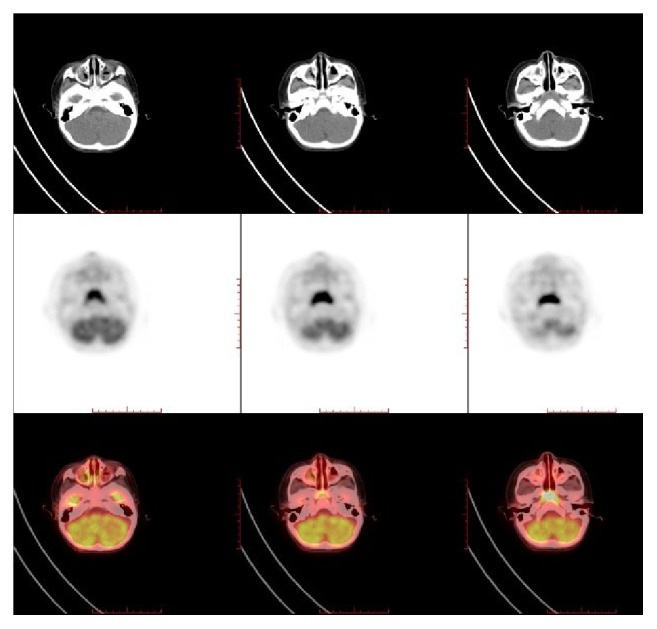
The normally increased uptake of ^18^F-FDG (SUV_max_ 11) in the nasopharynx of a 1-year-old boy is symmetric and trapezoid.
